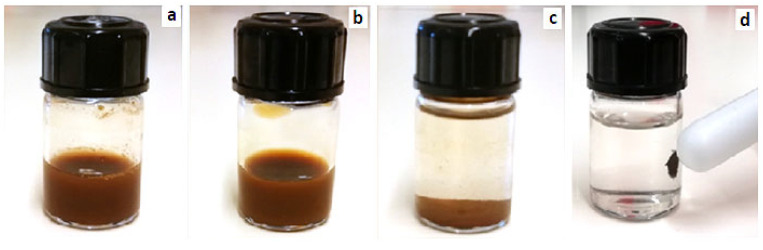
Citration steps of Fe_3_O_4_ NPs: (a) after the addition of deionized water to the magnetic NPs and dispersion in an ultrasonic bath, (b) after the addition of sodium citrate and placing it in the oven, (c) after the addition of acetone and precipitation of citrated magnetic NPs, and (d) the attraction of citrated magnetic NPs to the magnet.
